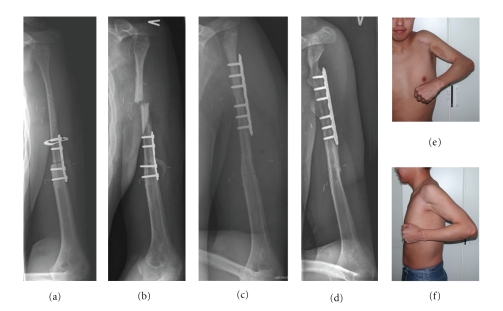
Resection of the proximal part of the humerus because of an osteosarcoma and reconstruction with a vascularized fibula graft including the proximal epiphysis (patient 5). X-rays taken 1 month postoperatively (a), and after 8 months when the junction was healed but the patient had just sustained a fracture of the graft (b) treated by plate ostesynthesis (c). 29 months after insertion of the graft, where the patient has had one more fracture just above the plate healed on conservative treatment; X-ray (d) and clinical photos showing active abduction (e) and extension (f) of the shoulder joint just before removal of the plate.
